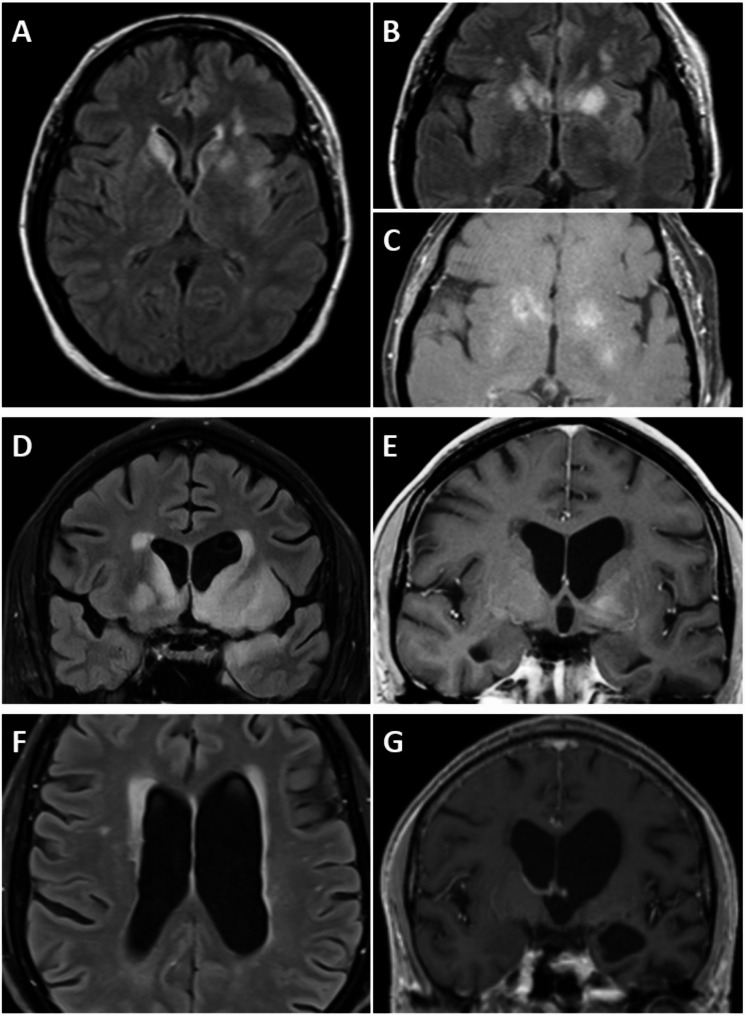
Magnetic resonance imaging (MRI) findings in two copine-5-IgG-positive patients. A-C MRI showing lesions in the basal ganglia (especially the heads of the caudate nuclei), paraventricular region, hippocampus, and frontal lobe in patient 1, two weeks after clinical onset, on axial (A) and coronal (B) T2/FLAIR imaging, with gadolinium (Gd) enhancement in the basal ganglia notable on coronal T1 imaging (C). D-E Coronal MRI imaging in patient 2 showing predominantly left-sided, extensive hyperintensities extending from the basal ganglia to the temporal lobe in T2 dark fluid imaging (D) with mild Gd enhancement in the basal ganglia (greater on the left than on the right) on T1 imaging 3 months after neurological onset (E). F (axial) and G (coronal): Periventricular T2 dark fluid hyperintensities and dilatation mainly of the left-sided lateral ventricle in patient 2 at month 6 (F; no coronal sequences available) and marked brain atrophy with dilatation of mainly the left lateral ventricle at month 21 on T1/Gd-weighted imaging (G)
